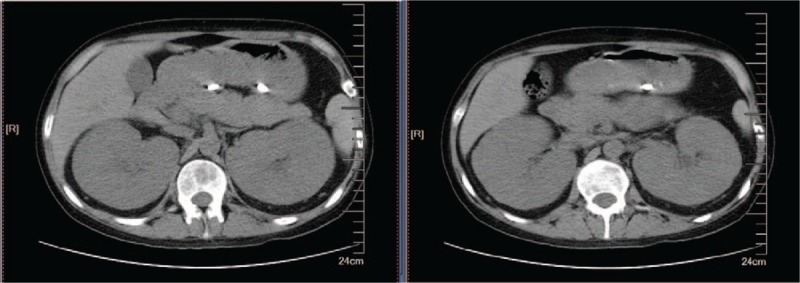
Abdominal computed tomography showing bilateral symmetrical, low-density, homogeneous renal enlargement.
